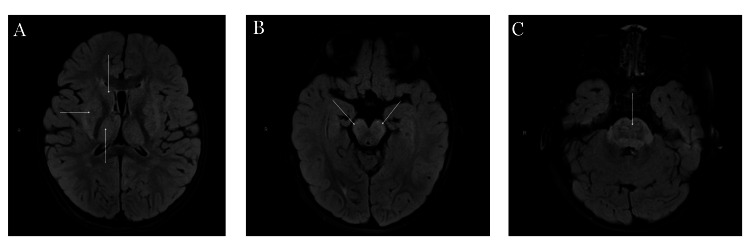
Representative axial magnetic resonance imaging (MRI) sections on fluid-attenuated inversion recovery (FLAIR). (A) Representative axial MRI sections highlight extensive signal abnormalities involving the bilateral thalami, putamen, and caudate nuclei, most conspicuous on post-contrast FLAIR imaging. (B, C) Additional extensive signal abnormality involves the brainstem, including the pons and lower midbrain, with extension into the ventrolateral medulla and the middle and superior cerebellar peduncles.
